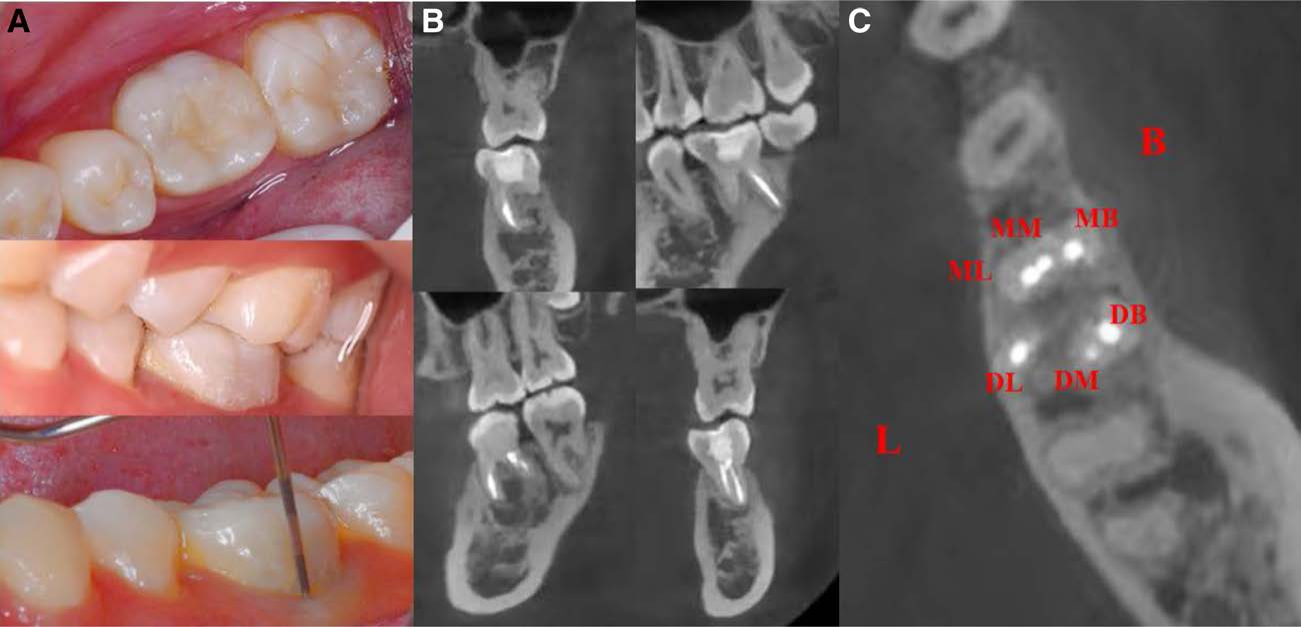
Intraoral photographs and CBCT images of tooth 36 at the 6-month follow-up visit. (A) Intraoral photographs, (B–C) CBCT images. CBCT = cone-beam computed tomography.
